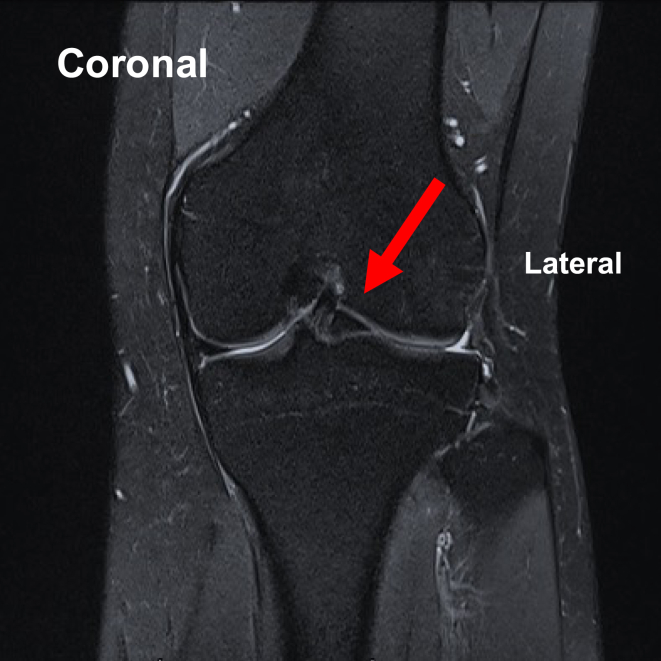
Preoperative coronal magnetic resonance imaging showing a bucket-handle tear of the lateral meniscus (arrow) in the left knee.
